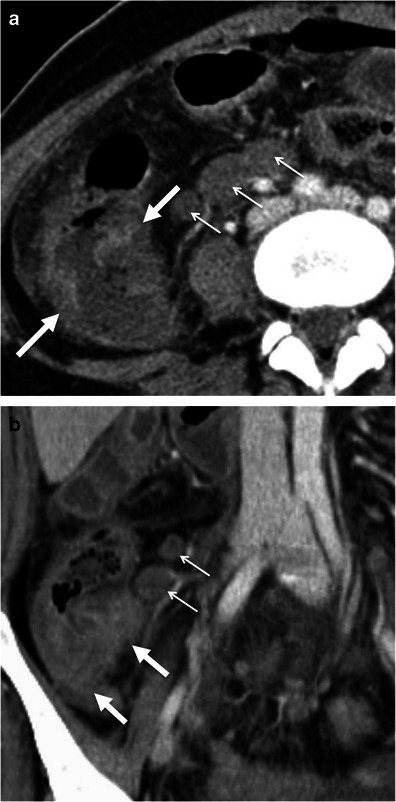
Intestinal tuberculosis. Axial (a) and reformatted coronal (b) contrast-enhanced CT scans show parietal irregular and asymmetric thickening of the caecum (*large arrows*), an appearance that mimics colon cancer. Also note low attenuation adenopathy (*thin arrows*), a usual finding in tuberculosis. Mild pericolonic fat stranding is also seen. *Adapted from the electronic poster “Bowel wall thickening—a complex subject made simple” DOI:*10.5444/esgar2011/EE-063
